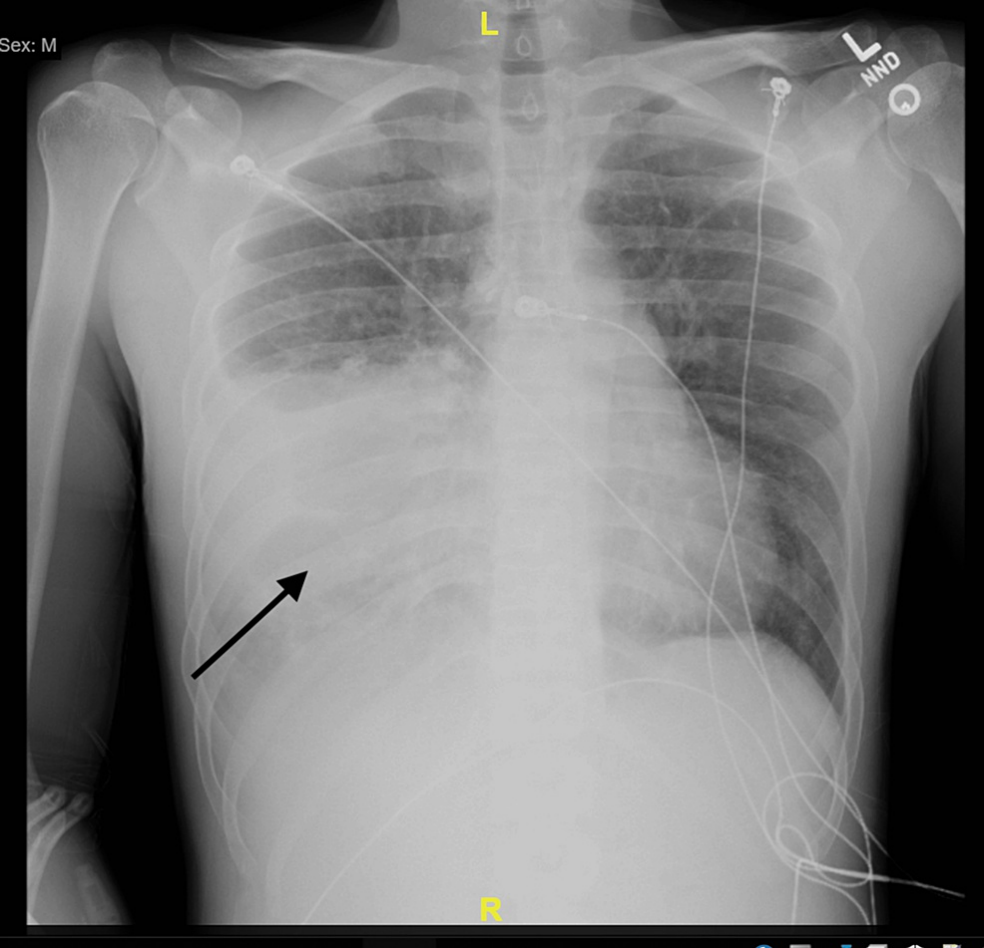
Chest radiography with right lower lobe infiltrates
